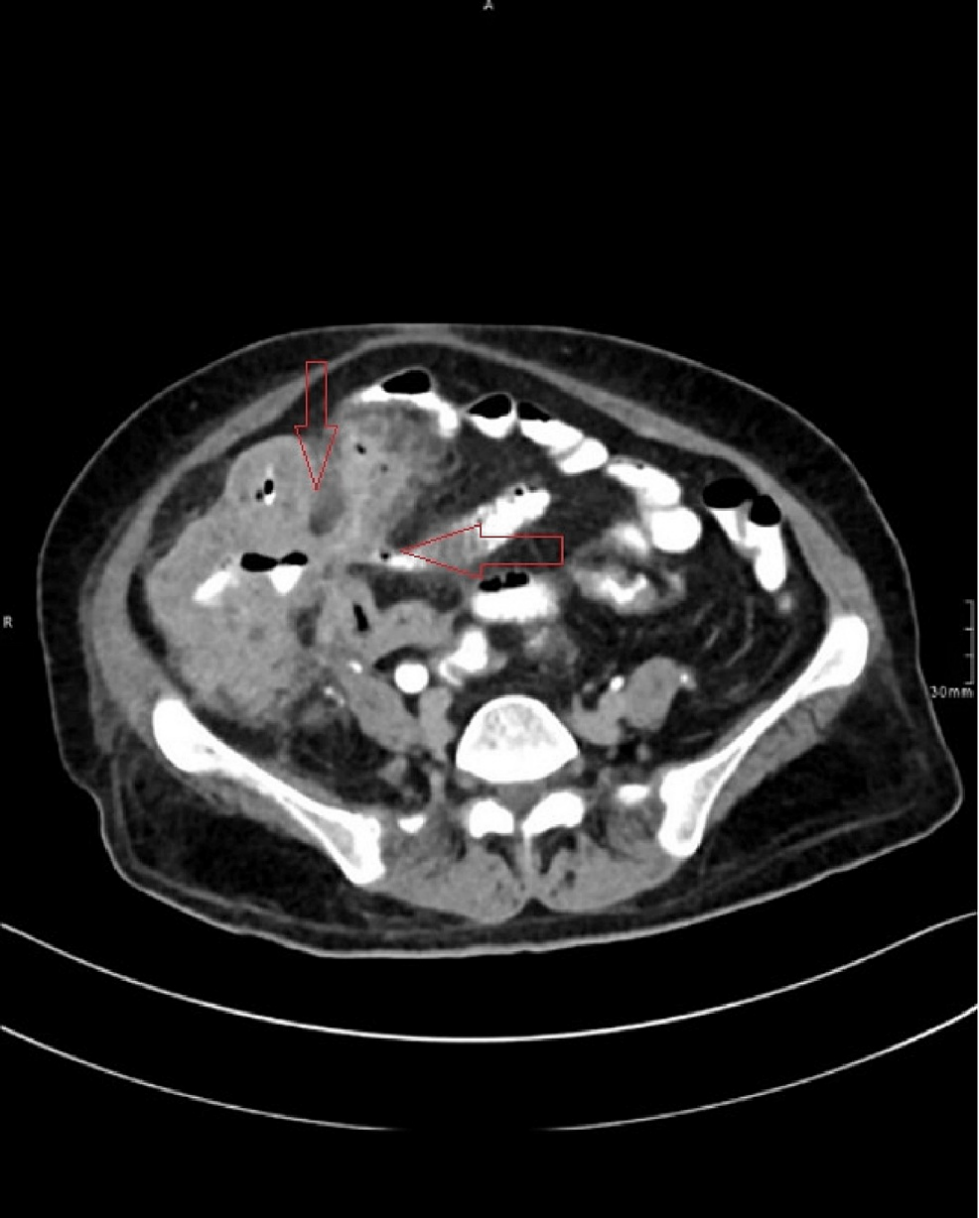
CT of the abdomen showing an entero-colonic fistula CT: computed tomography
